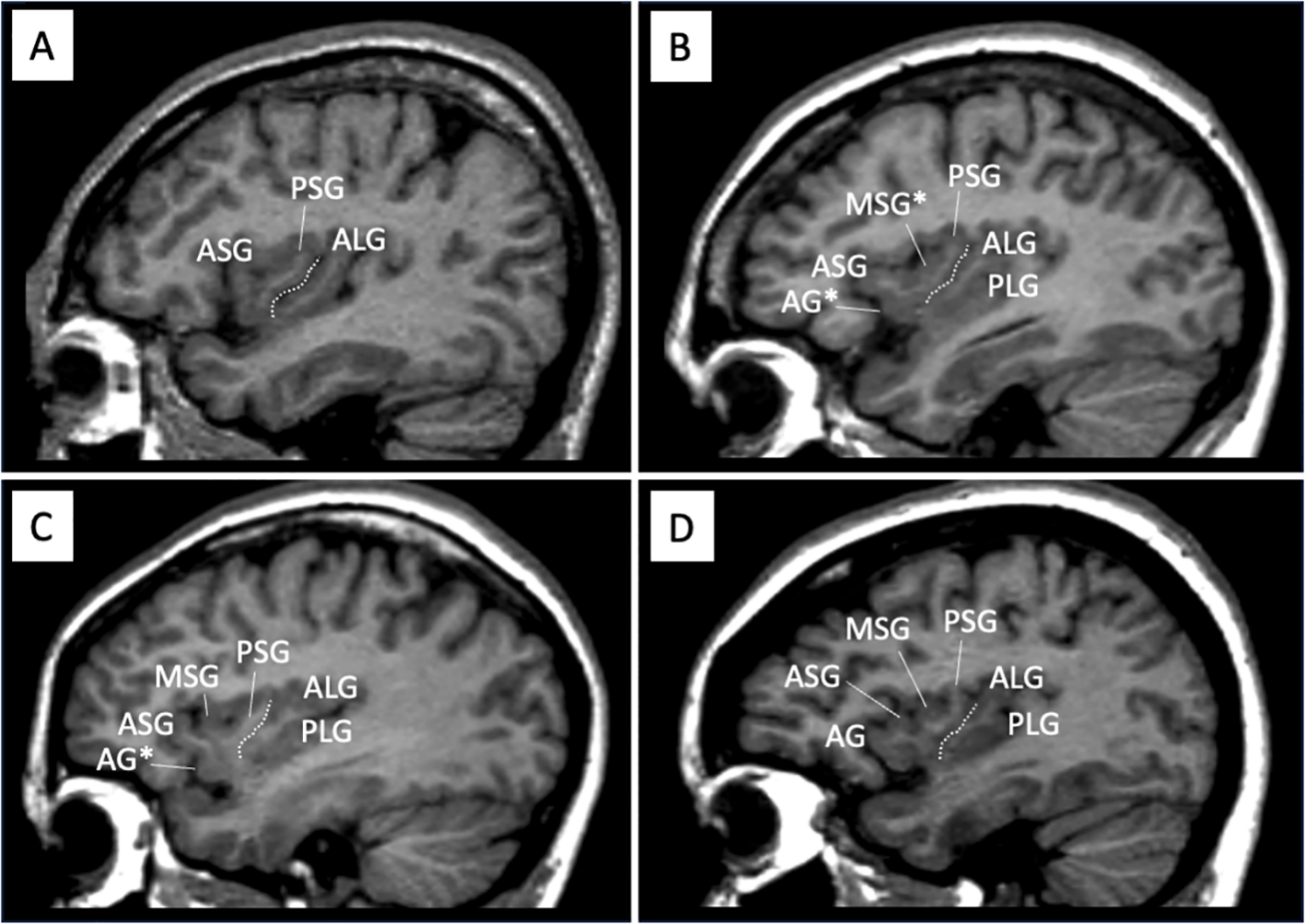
Sample sagittal MR images of different gross insular patterns. The developmental pattern of each gyrus was assessed predominantly using consecutive sagittal slices, with also coronal and axial views simultaneously being referred to. Dotted lines indicate the location of the central insular sulcus that subdivides the insula into the anterior (short) and posterior (long) cortices. The ASG, PSG, and ALG were well-developed in all participants in this study, while the PLS was sometimes deficient panel (A). The AG and MSG in the short insula had a large inter-individual variation, where the gyrus was absent panel (A), underdeveloped [marked with asterisks in panels (B, C)], or well-developed panel (D). AG, accessory gyrus; ALG, anterior long gyrus; ASG, anterior short gyrus; MSG, middle short gyrus, PLS, posterior long gyrus; PSG, posterior short gyrus.
